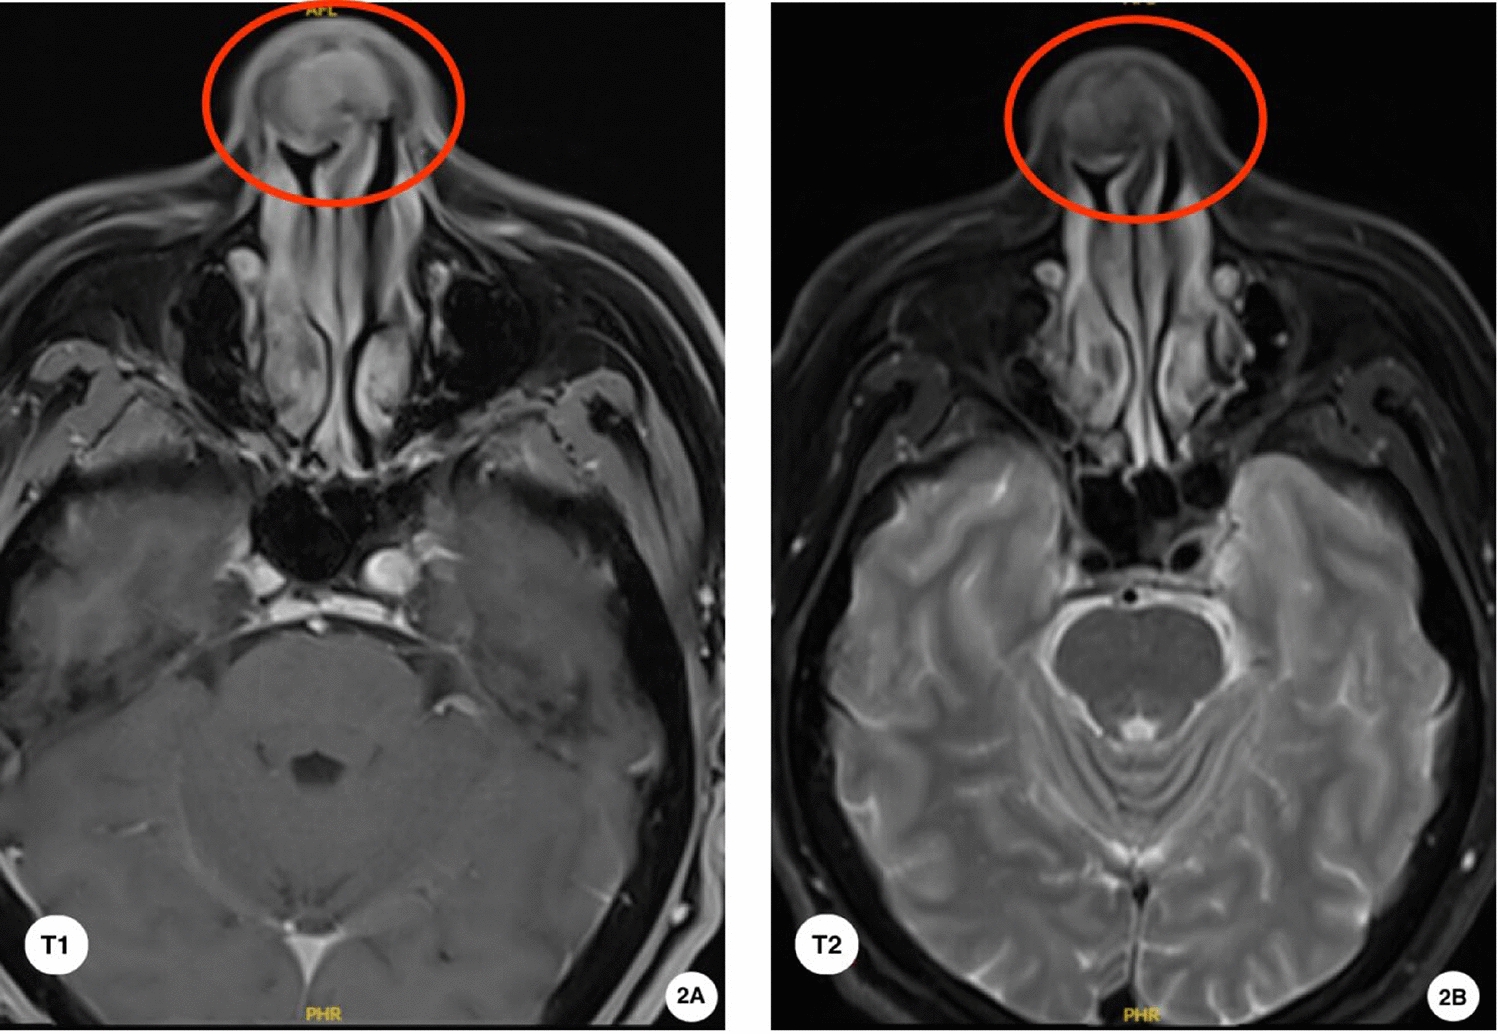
Nasal septum magnetic resonance imaging (axial view) findings. A T1 post-contrast image shows an enhancing hypointense nodule within the nasal septum. B T2-weighted image demonstrates a nasal septal nodule with heterogeneous signal intensity (mixed high and low signal). The red circles indicate the location of the lesion (gouty tophus) in the nasal septum
